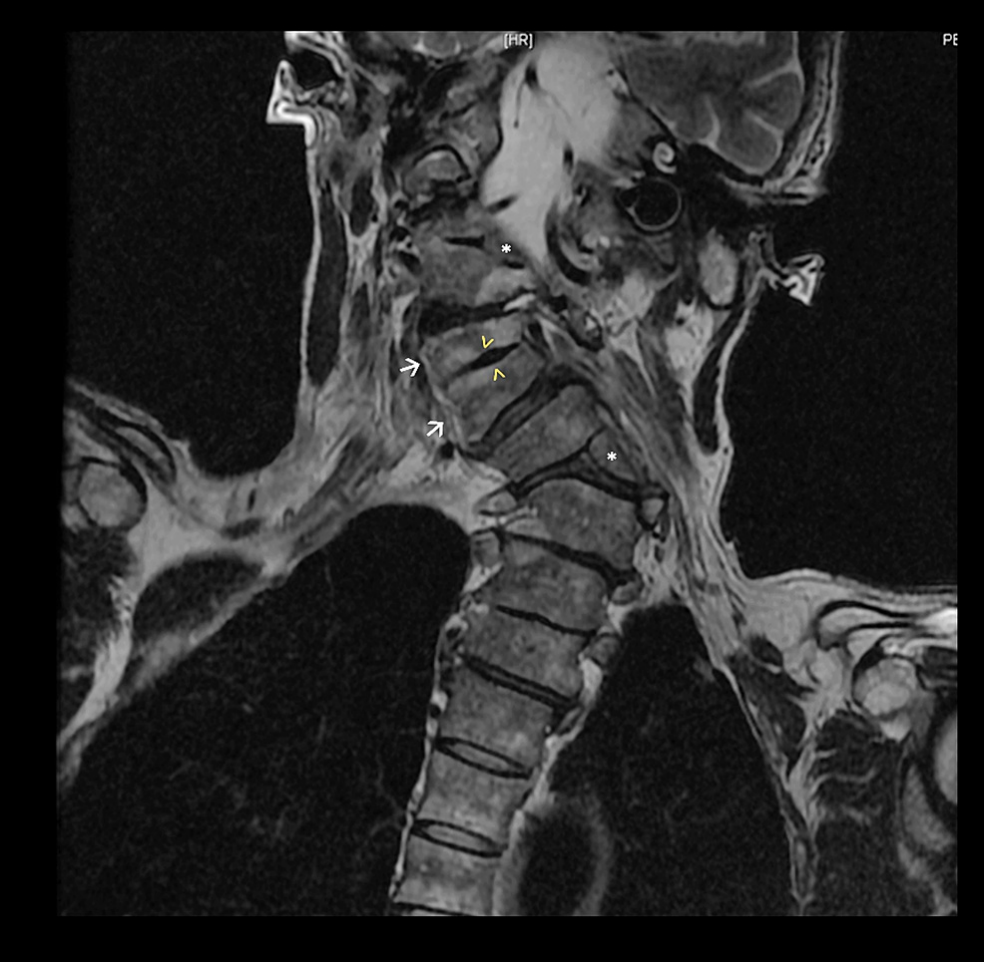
Cervical MRI, coronal plane, T2-weighted image Cervical MRI showed no signs of fracture. Complete atlanto-occipital assimilation is observed. There is a left C3 hemivertebra (upper asterisk), not segmented, associated with partial fusion in the right lateral portion of C2 and C4. There is a C5-C6 block vertebra (white arrows). The intervertebral disc between these two vertebrae is degenerated (arrowheads) and partially absent, and there is an incomplete bone fusion. There is another hemivertebra, presumably T1 (lower asterisk).
